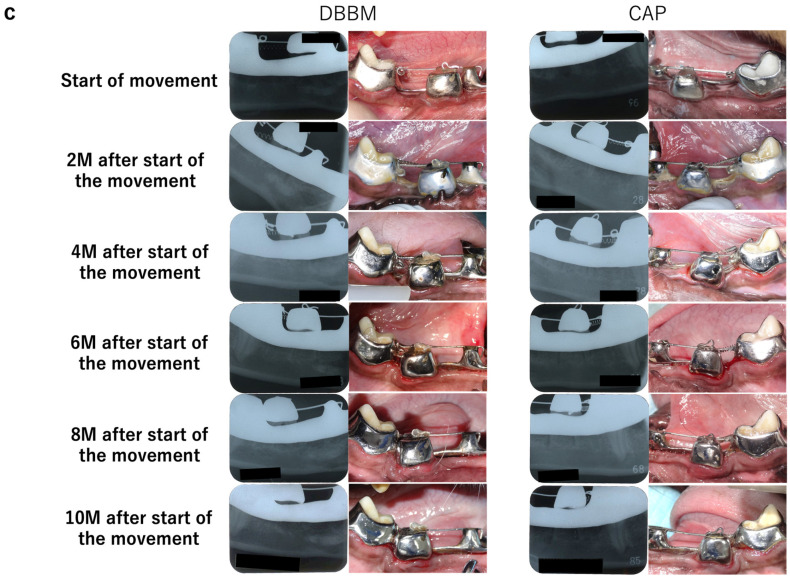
Intraoral photographs and dental radiographs obtained during tooth extraction and bone filler grafting. (a) Intraoral photograph. Tooth extraction and carrier implantation were performed. The state of healing 3 months after transplantation and a photograph of the attached device are shown. (b) Dental radiographs at the time of transplantation and 1-, 2-, and 3 months post-transplantation. DBBM was implanted on the right side, and CAP was implanted on the left side. Two months after the transplantation, trabecular formation was observed. (c) Intraoral and dental X-ray photographs every 2 months after the movement started.
